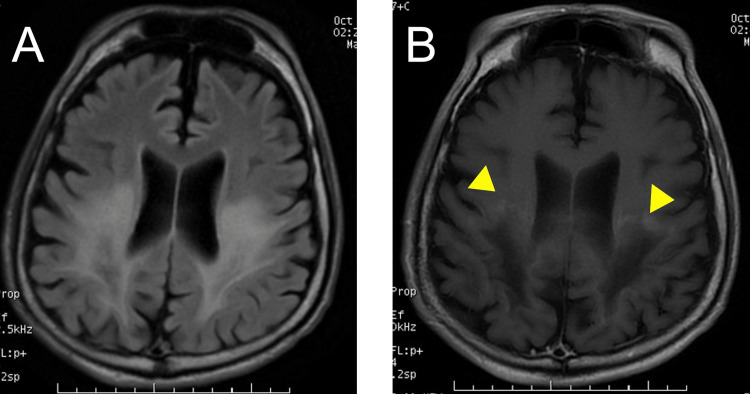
Brain MRI findings in patient 1 (A) Fluid-attenuated inversion recovery (FLAIR) imaging shows a diffuse high-intensity lesion in deep white matter in the parieto-occipital lobes. (B) Contrast-enhanced T1-weighted imaging shows border enhancement in the anterior periphery of the lesion demonstrated by the FLAIR image (yellow arrowheads)
